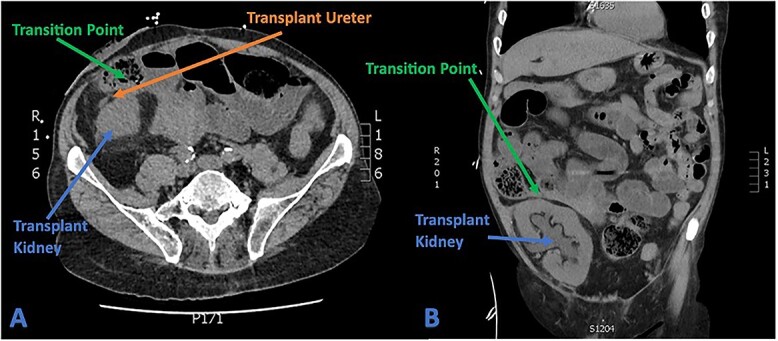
(A) Axial CT section showing transplant ureter coursing anterior to kidney transversing over the transition point of SBO. (B) Coronal CT section showing faecalized small bowel approaching transition point with gross hydroureter and hydronephrosis of transplant kidney.
